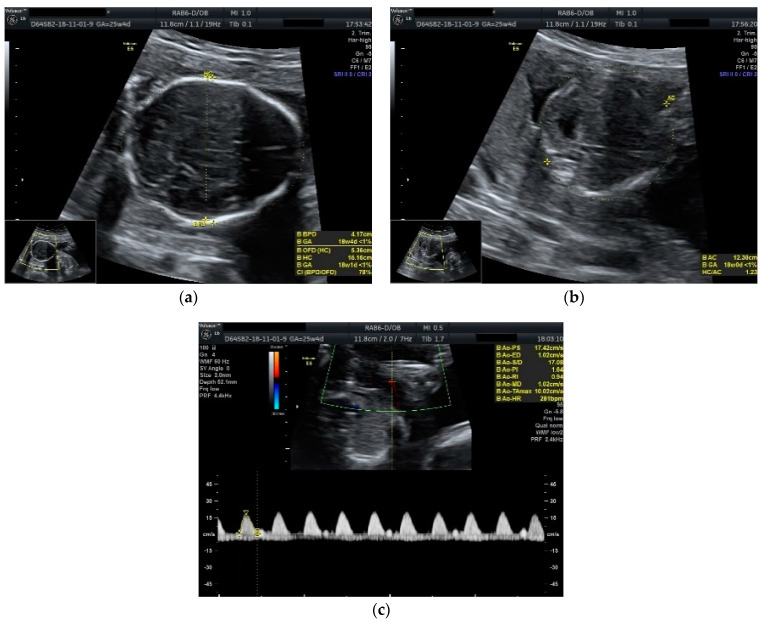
Ultrasound scan performed at 25 weeks and 4 days of gestation: (a) biparietal diameter of the growth restricted fetus appropriate for 18 weeks and 4 days of gestation; (b) abdominal circumference of the growth restricted fetus appropriate for 18 weeks and 0 days of gestation; (c) umbilical artery Doppler scan of the growth restricted fetus showing the absence of diastolic flow.
